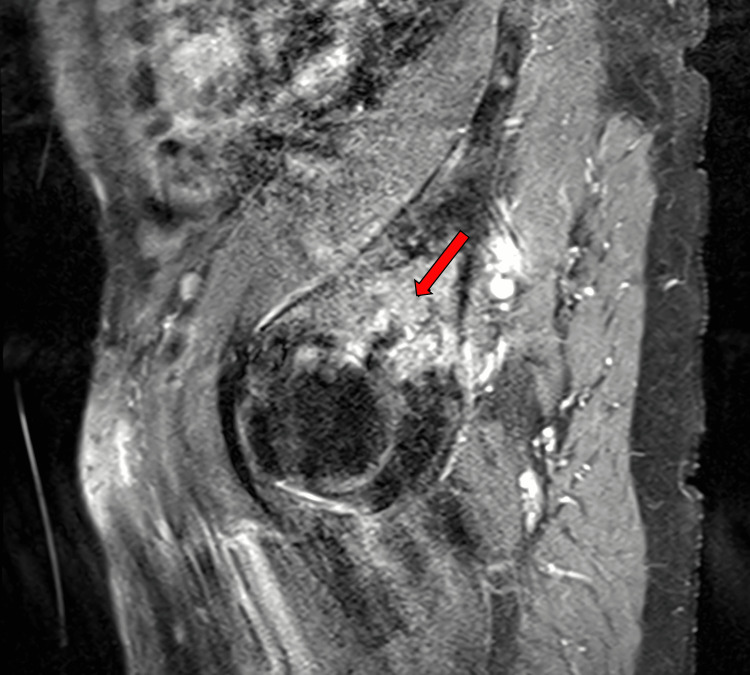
T1-weighted pelvic MRI with contrast, sagittal view An expansive enhancing lesion consistent in location, with the initial mass seen on a CT scan measuring greater than 6 cm in diameter, which is notably larger than originally able to be measured on the CT scan, is present within the superoposterior acetabular roof of the left bony pelvis involving both iliac and ischial segments of the hemipelvis (arrow). Cortical thinning is also present and indicates the potential for a pathologic fracture with unassisted ambulation. The full image series reveals smaller enhancing lesions within the sacrum, iliac wings, and right femoral neck.
